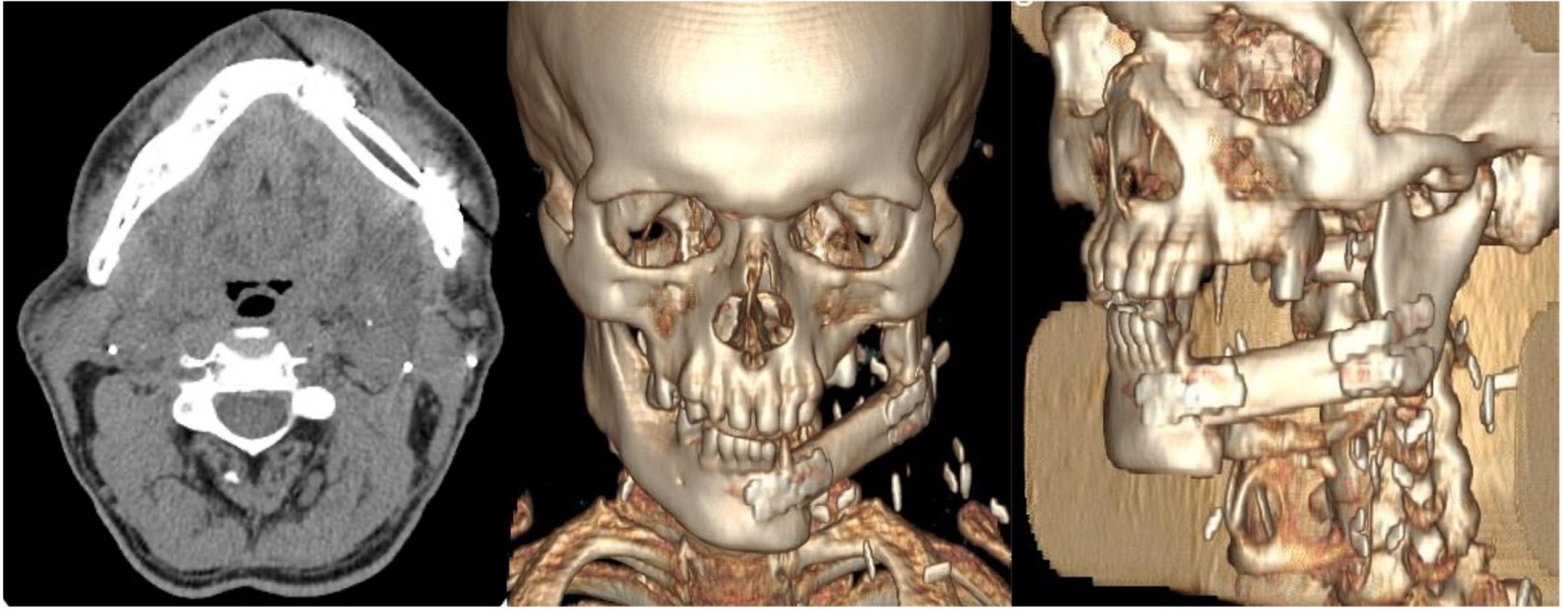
Post-operative CT scans of the patient showing correct placement of the free fibula flap to reconstruct the left hemimandibular defect. The flap is secured with miniplates to the residual mandibular segments and positioned superiorly at the level of the alveolar crest to ensure optimal future implant rehabilitation.
